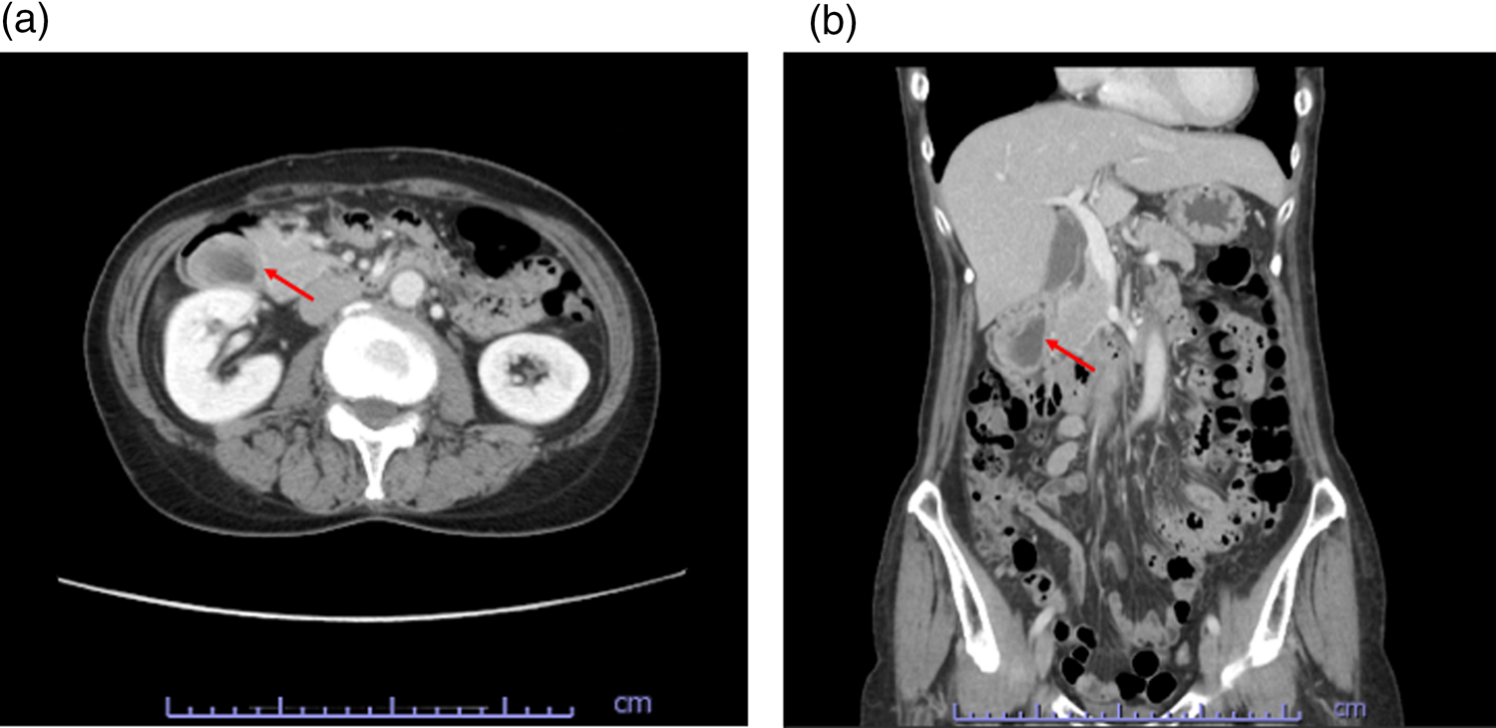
Abdominal computed tomography findings computed tomography demonstrated (red arrows) a 40‐mm pedunculated mass with internal nonuniformity in the descending part of the duodenum. (a) coronal image and (b) axial image
